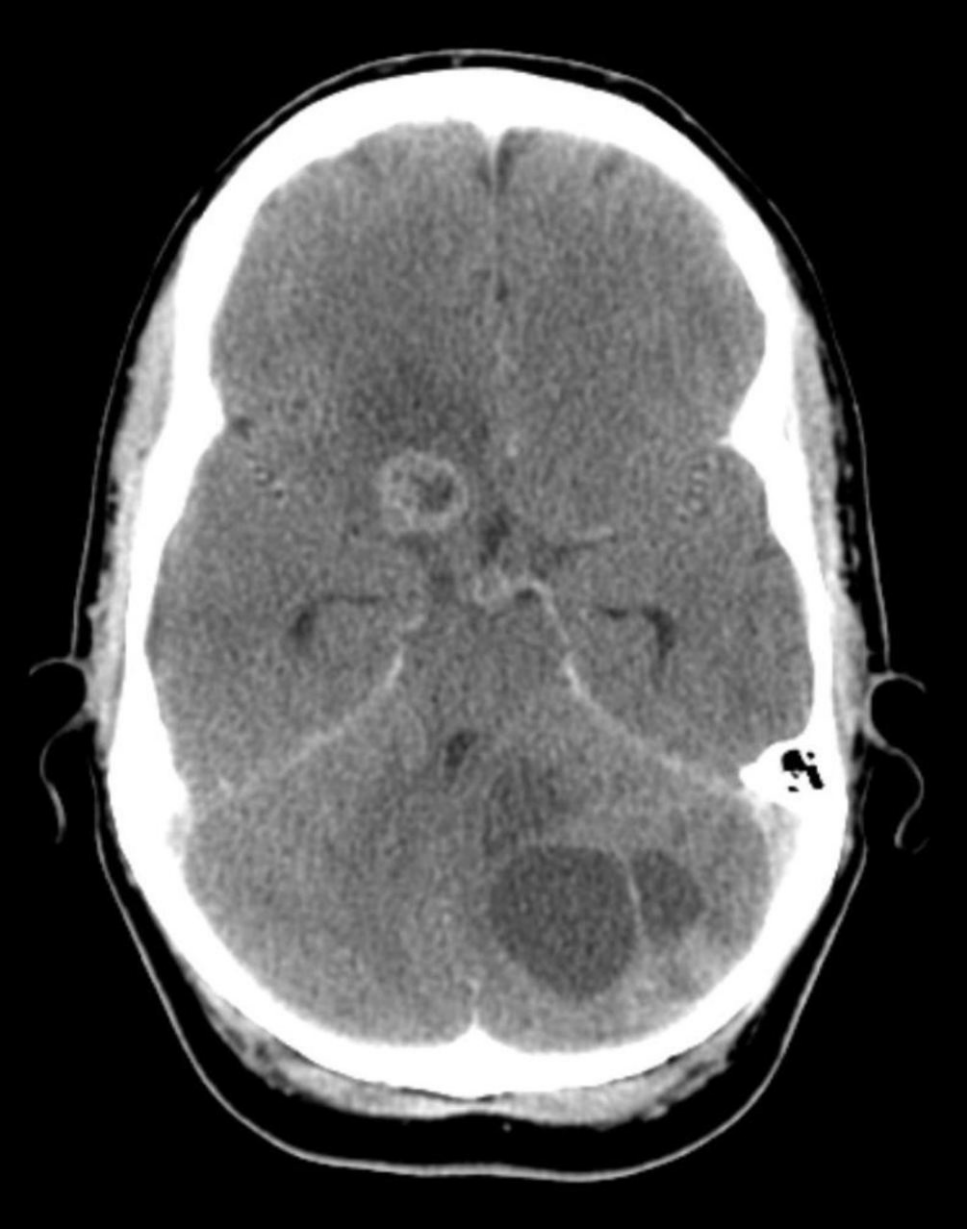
Brain metastases (computed tomography)
